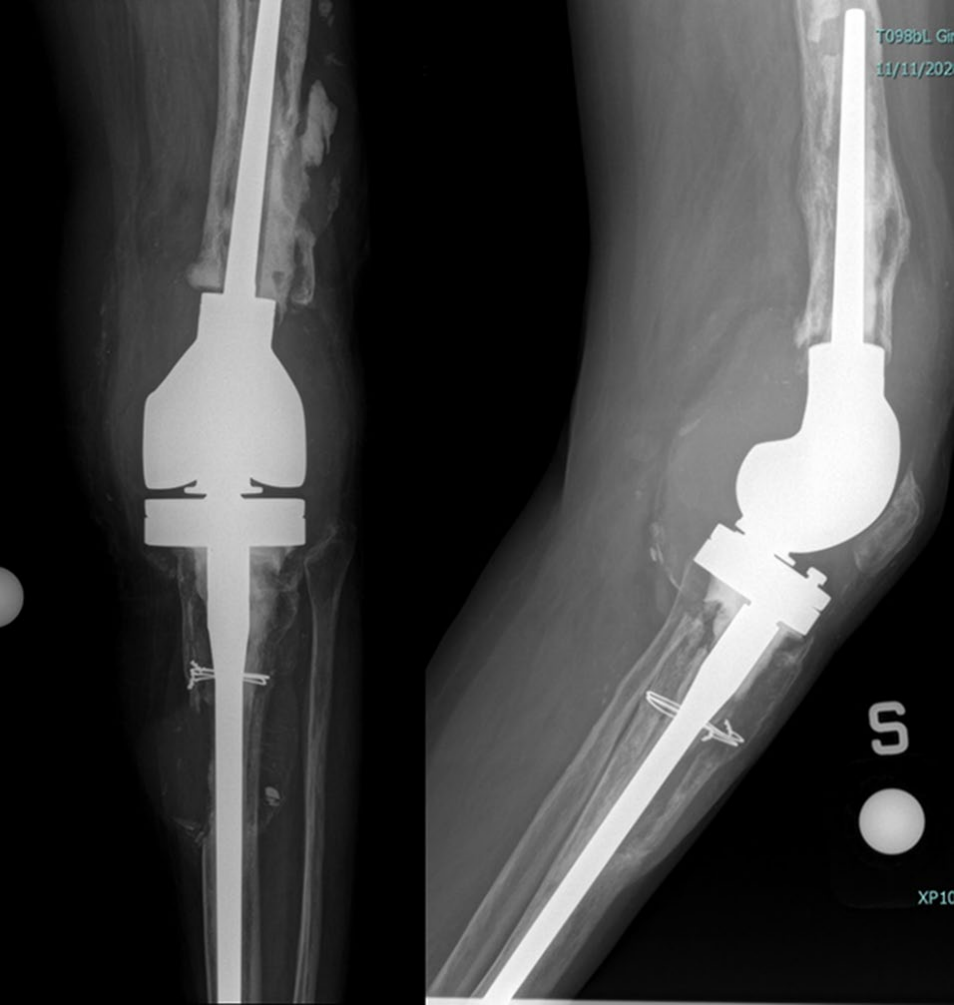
Preoperative X-rays: Aseptic loosening with severe osteolysis and pseudotumor formations around tibial component
